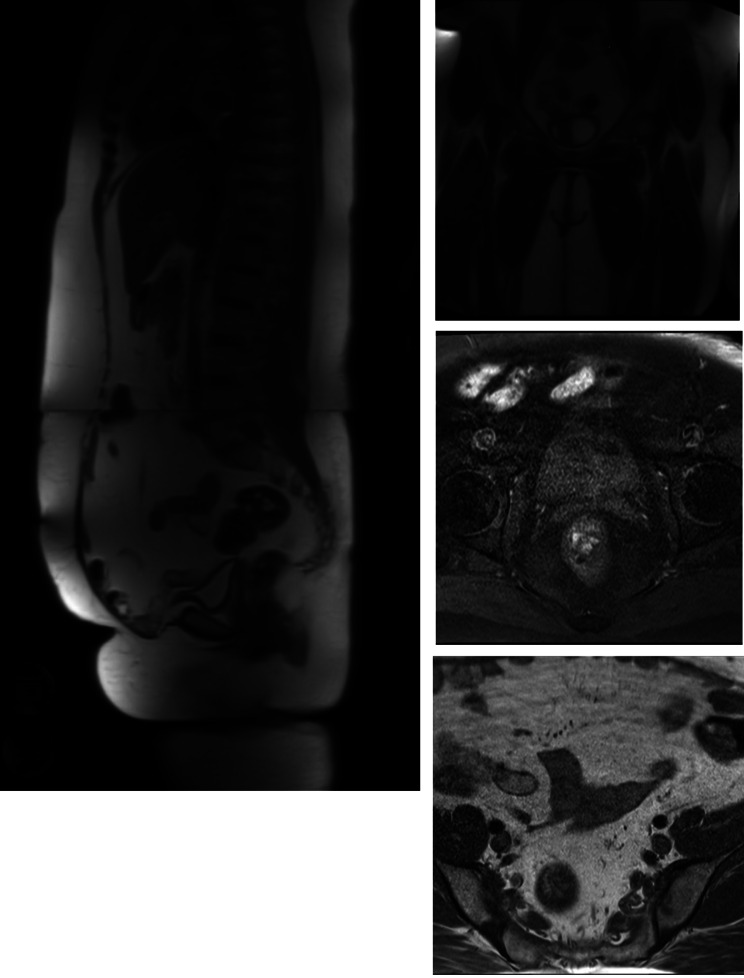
Post-surgical abdominal Magnetic Resonance Imaging. T1 Images in the sagittal plane, coronal plane, and axial plane of fat saturation and non-fat saturation
